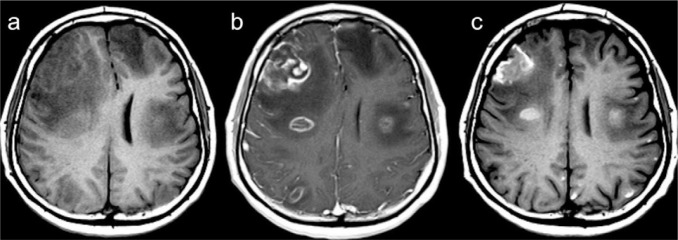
MRI scans of a patient with cerebral toxoplasmosis. Unenhanced axial
